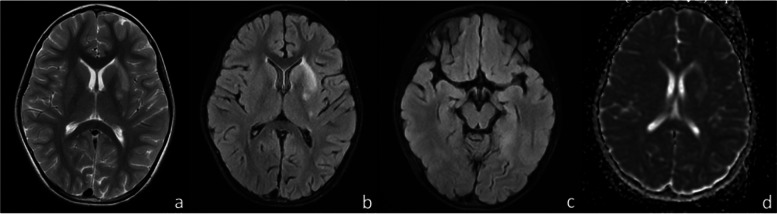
Head MRI (a T2WI axial image, b, c T2-FLAIR axial image, d DWI axial image) showed a slightly longer patchy T1 and slightly longer T2 abnormal signal in the left basal ganglia, T2-FLAIR image showed hyperintensity, and DWI image showed no diffuse limited hyperintensity in the skull. Hyperintensity patches on the left cauda equina, bilateral frontotemporal cortex, cingulate gyrus were observed on local T2-Flair with unclear boundaries, but no hyperintensity patches were observed on DWI. No enhancement was observed in the enhancement scan
